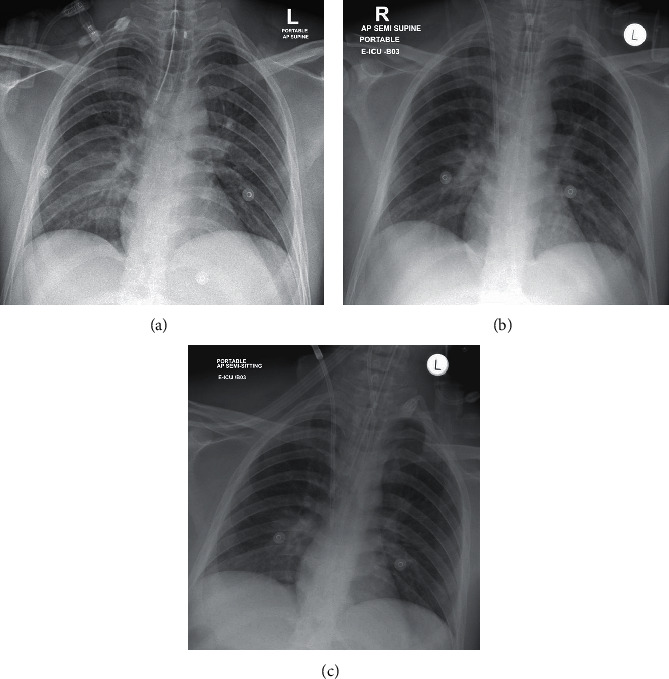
Repeat chest X-rays also did not show any classical findings for COVID-19.
